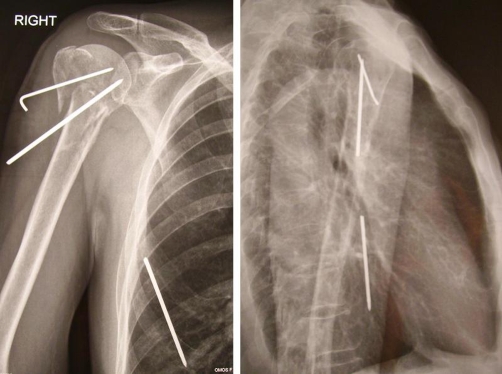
Intrathoracic migration of a Steinman wire; post-operative radiograph on the third week.
